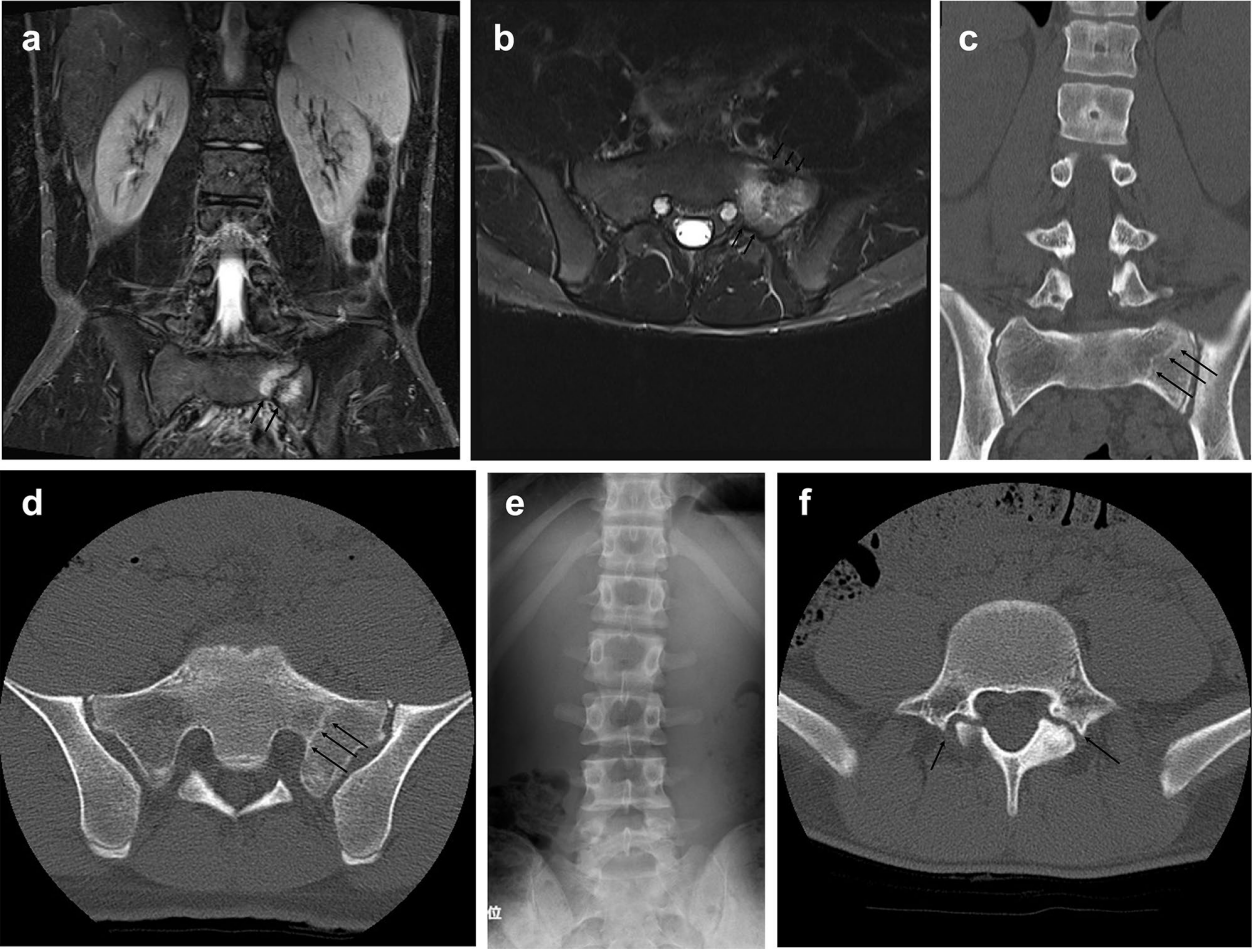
Pretreatment images of the representative case. (a) The MRI STIR coronal view shows a signal changed on the oblique line on the left sacral alar (arrows indicate a signal changed line). (b) The MRI STIR axial view shows a massive change in the signal on the left sacral alar (arrows indicate bone marrow edema). (c) CT coronal view showing a sclerotic oblique line on the left sacral alar (arrows indicate sclerotic line). (d) CT axial view of the sacrum shows a sclerotic oblique line on the left sacral alar (arrows indicate sclerotic line). (e) Plain radiograph AP view shows no abnormal changes. (f) CT axial view of the fifth lumbar shows pseudarthrotic bilateral spondylolysis (arrows indicate bilateral bony cleft).
